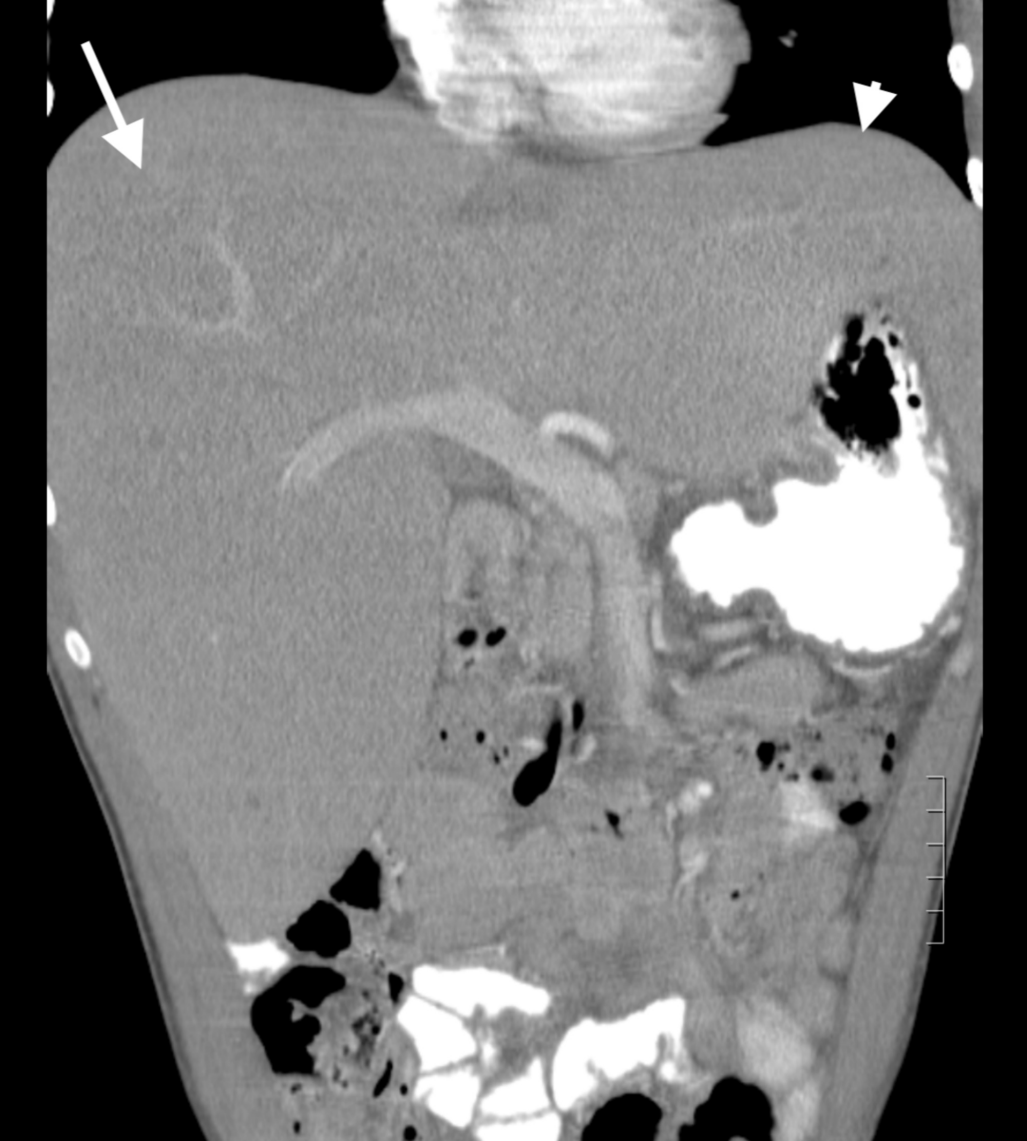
CT scan of the abdomen A coronal section of the abdominal CT scan showing massive hepatomegaly with the right lobe (white arrow) pushing down the bowel and the left lobe of liver extending up to the left upper quadrant of the abdomen (white arrowhead).
